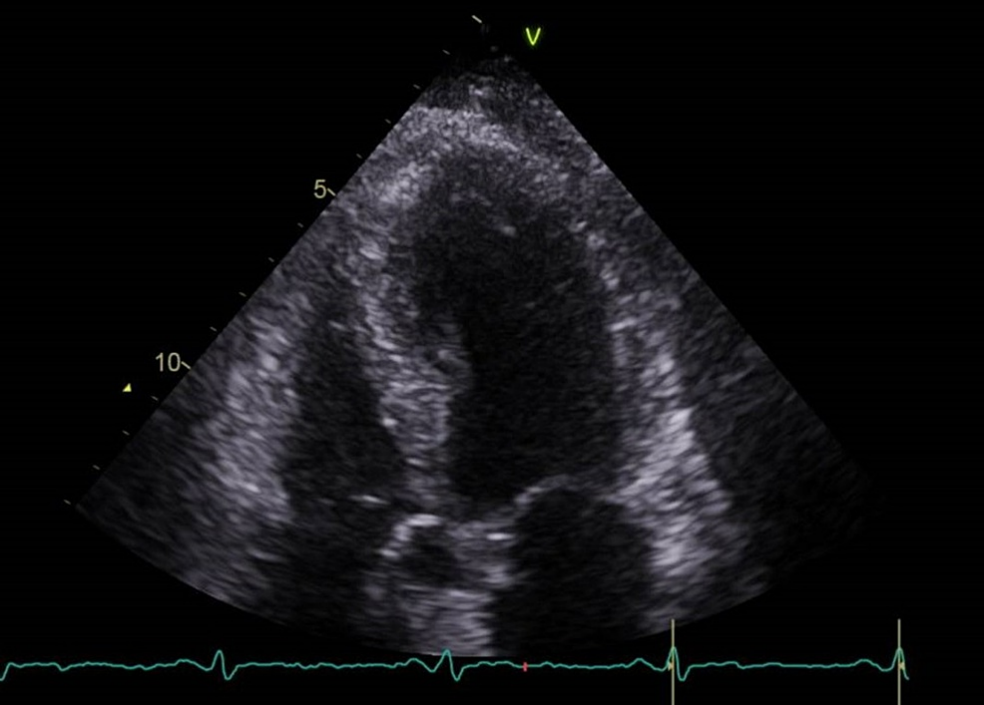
Transthoracic echocardiogram (modified 4 chamber view with a left ventricle close up) reveals some ballooning of the left ventricle (LV) apex.
